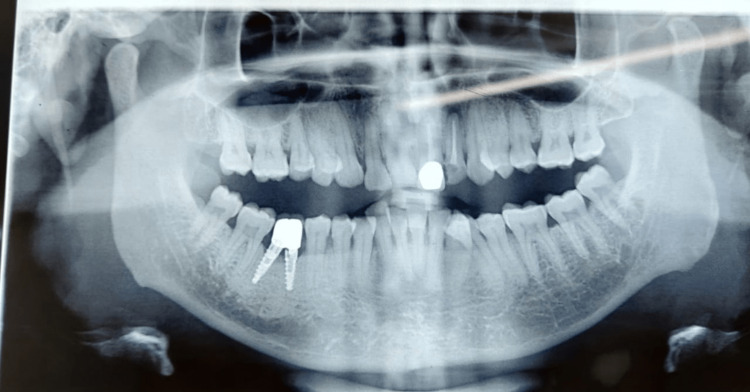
Postoperative orthopantomogram showing completed 46 (right mandibular first molar) dual screw-retained implant crown
